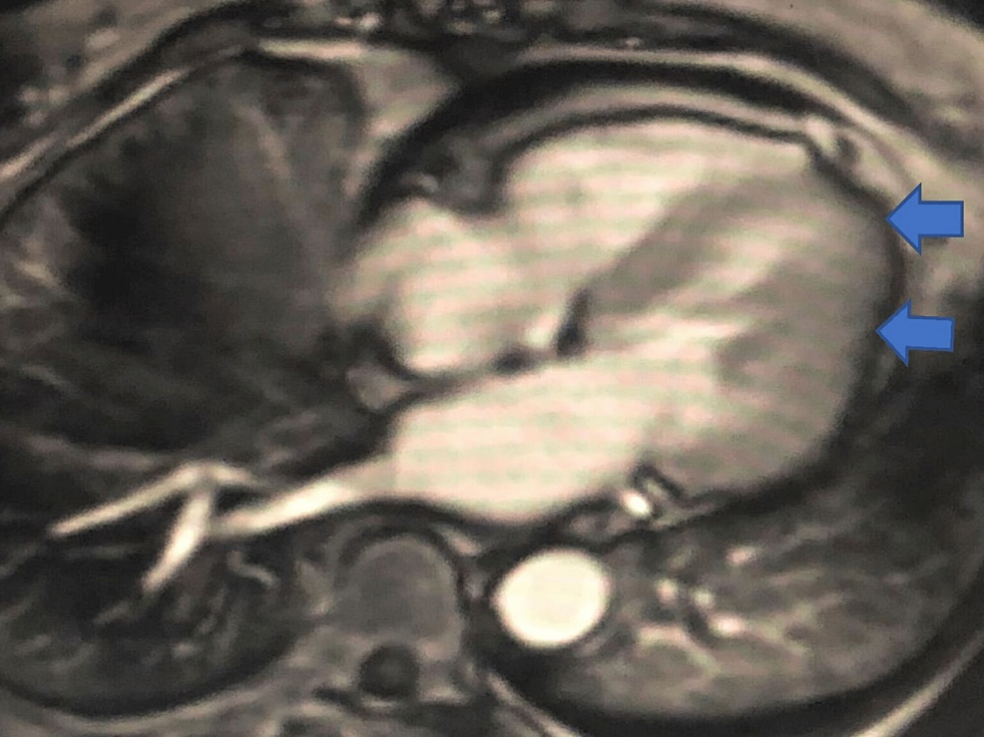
Cardiac magnetic resonance imaging 4 chamber view showing myocardial oedema and fibrosis suggestive of an acute inflammatory process as shown by blue arrows
